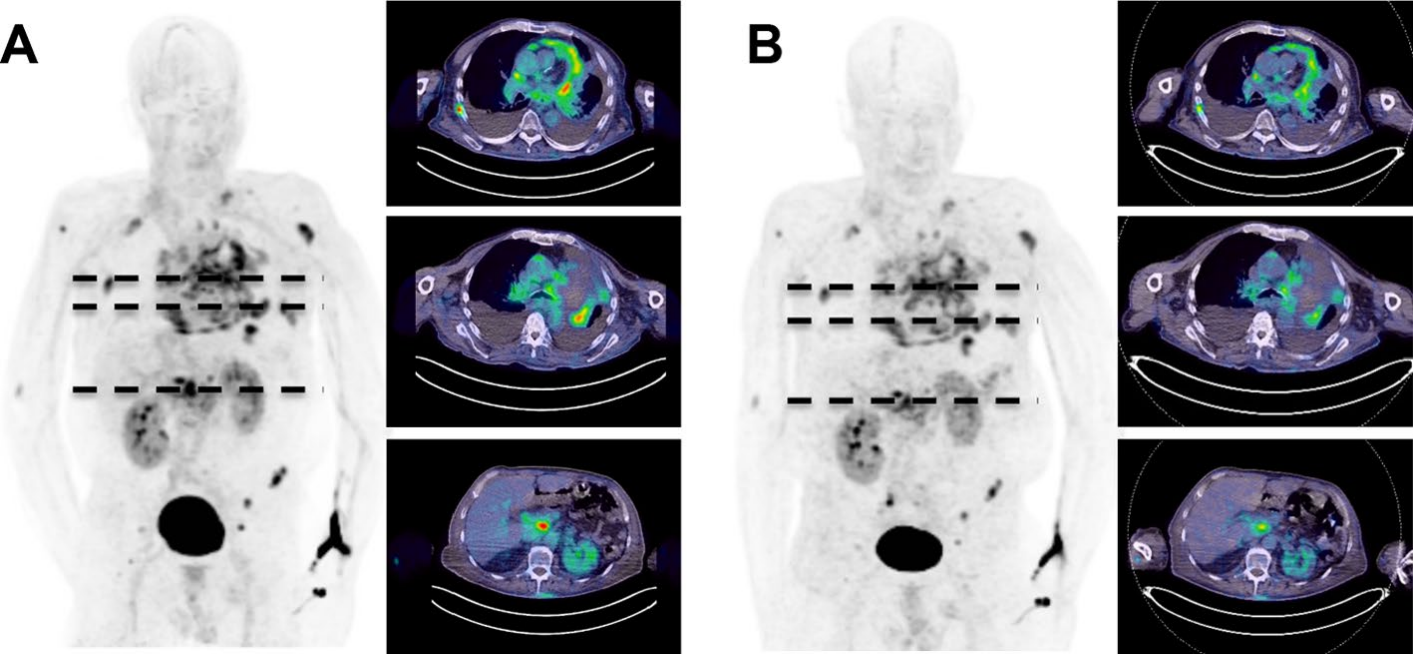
Whole body PET/CT images of a 68y old patient with NSCLC in the left upper lobe. Shown are the maximum intensity projections and transaxial cuts at A 1 h and B 3 h after administration of 323 MBq [^18^F]AlF-FAPI-74
